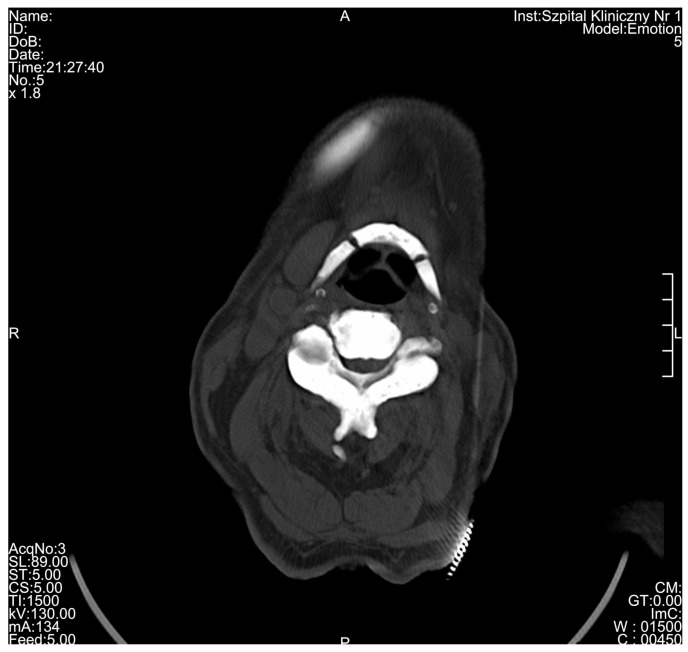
Significant osteosclerosis of the hyoid and cervical vertebra in 80-year-old patient suffering from osteopetrosis visible on cross-sectional computed tomography scan.
